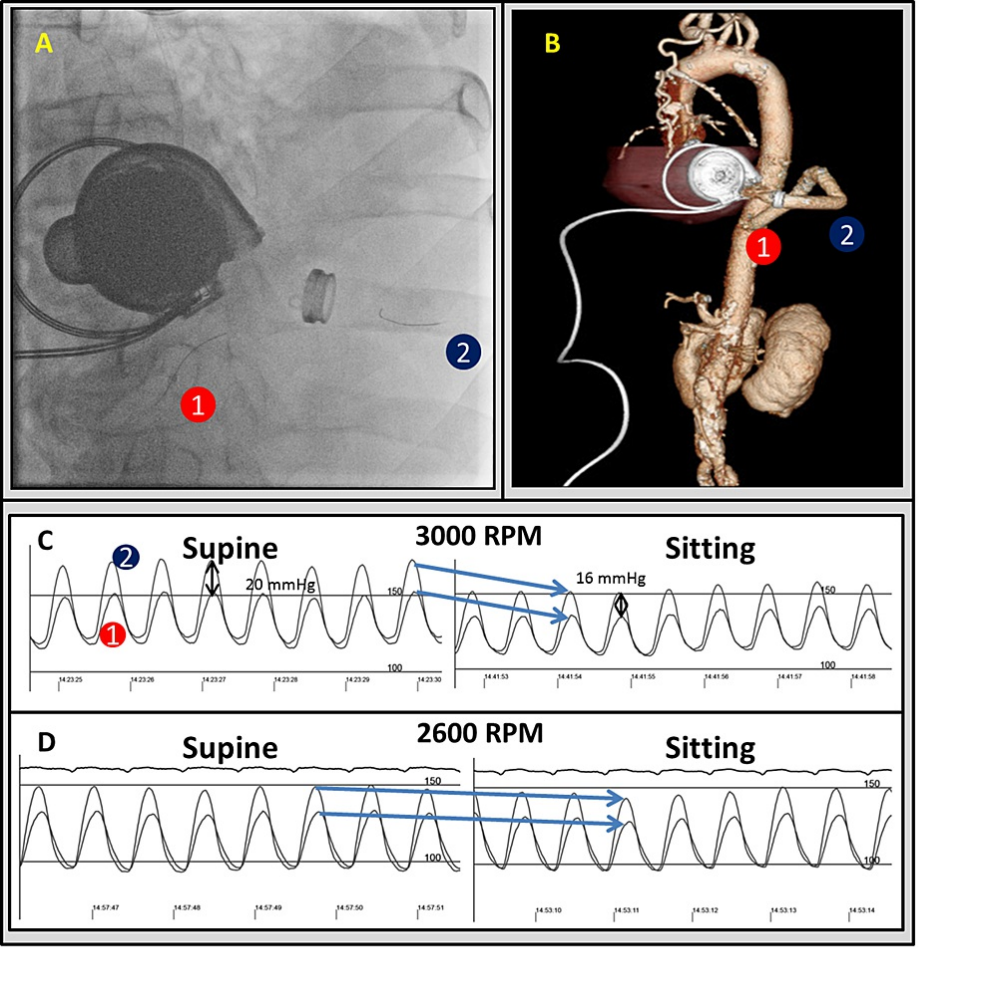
Hemodynamic evaluation of outflow and dynamic inflow obstruction Fluoroscopic image showing the location of the aortic catheter and high-fidelity micromanometer catheter in the proximal outflow graft (A) with corresponding CT locations (B). Hemodynamic waveforms showed dynamic obstruction with sitting at 3000 RPM with a reduced outflow gradient (C), whereas without dynamic inflow obstruction at 2600 RPM, there was only residual fixed obstruction in the outflow cannula and no evidence of a flow-mediated augmentation in gradient due to unkinking of the outflow graft (D). RPM - revolutions per minute.
